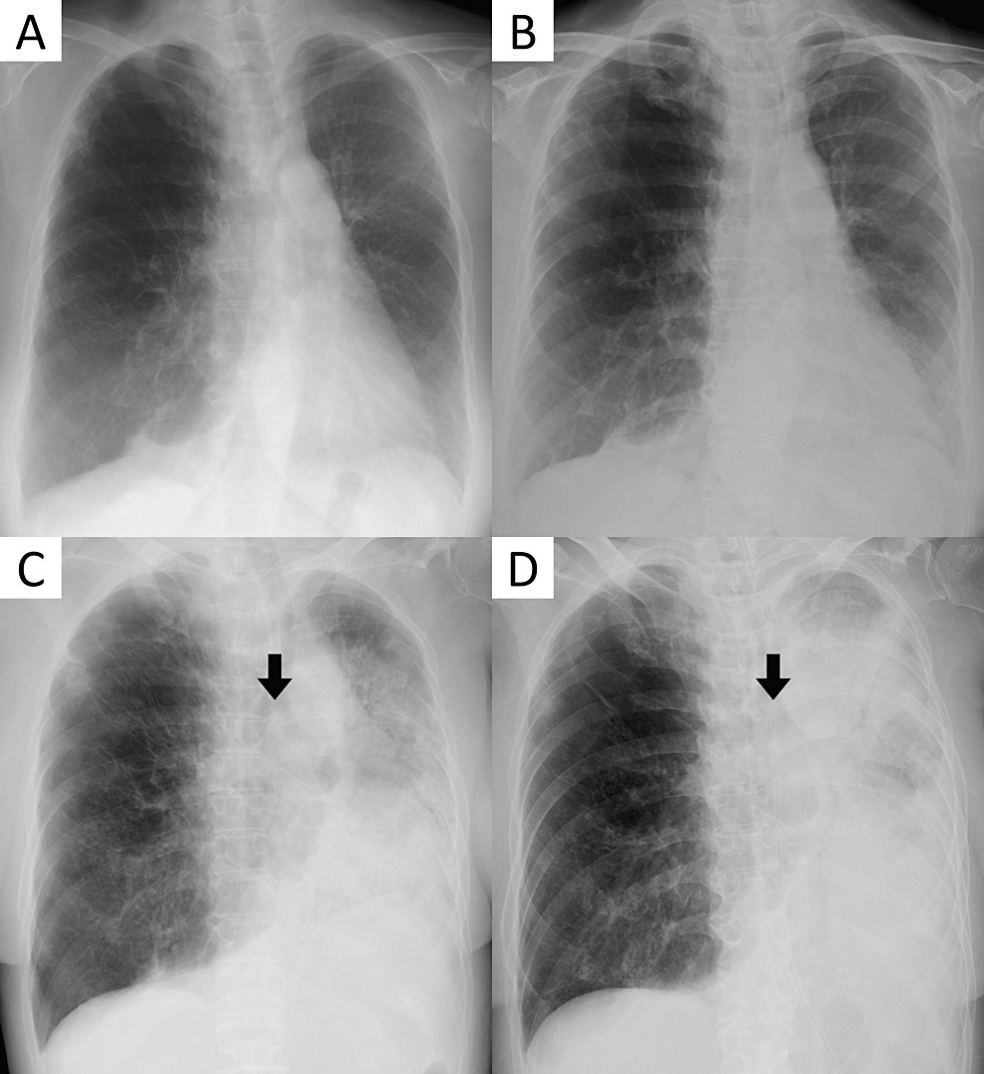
Chest x-ray findings of the patient. (A) Baseline chest x-ray after LTx. The right lung has a large volume and decreased density due to lymphangioleiomyomatosis, and there is no pulmonary infiltrate in the transplanted left lung, (B) diagnosis of COVID-19 pneumonia with increased density in the left middle and lower lung, (C) on the second admission to our hospital - 18 weeks after her COVID-19 diagnosis and 10 weeks after her dyspnea worsened - the pulmonary infiltrates in the left lung progress and mediastinal deviation appears (arrow), and (D) despite the steroid pulse, the left transplant lung opacification, volume loss, and mediastinal deviation (arrow) get worse. LTx: lung transplantation; COVID-19: coronavirus disease 2019
